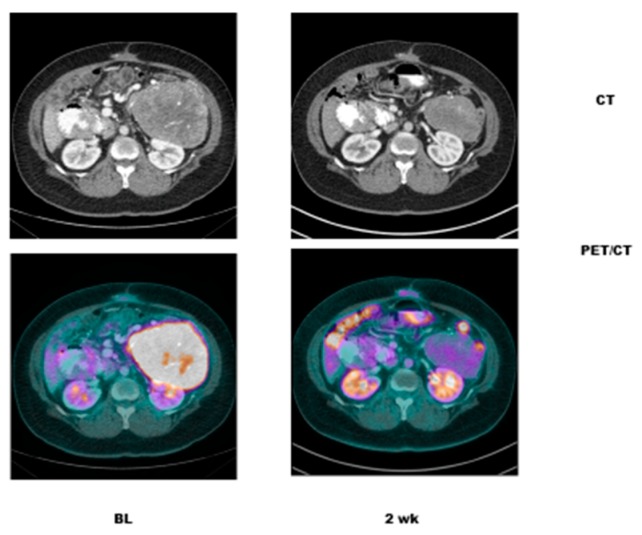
PRE and POST CT and PET/CT in a female in her 40’s with recurrent GIST. She has had multiple prior therapies and had relapsed from several TKI’s. After 2 weeks of novel therapy, she has had a complete metabolic response, but only a partial anatomic response.
